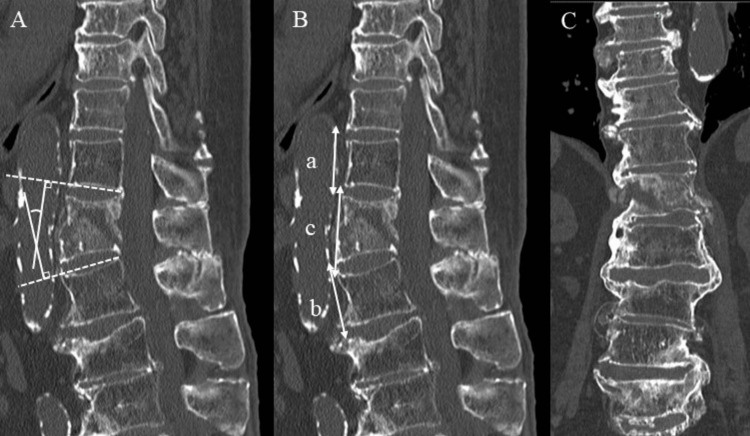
Image evaluation in CT A. Local kyphosis angle (°); B. Ratio of the anterior wall height (%); C. Plain CT coronal section The formula for the ratio of the anterior wall height is shown. A value closer to 100% indicates that the vertebral height of the affected vertebrae is reduced closer to its original height. The ratios of the anterior wall height of the cephalocaudal adjacent vertebral body to the fractured vertebrae with the adjacent craniocaudal disc height (a & b) and the anterior wall height of the fractured vertebral body with the adjacent craniocaudal disc height (c) are measured using this image.
